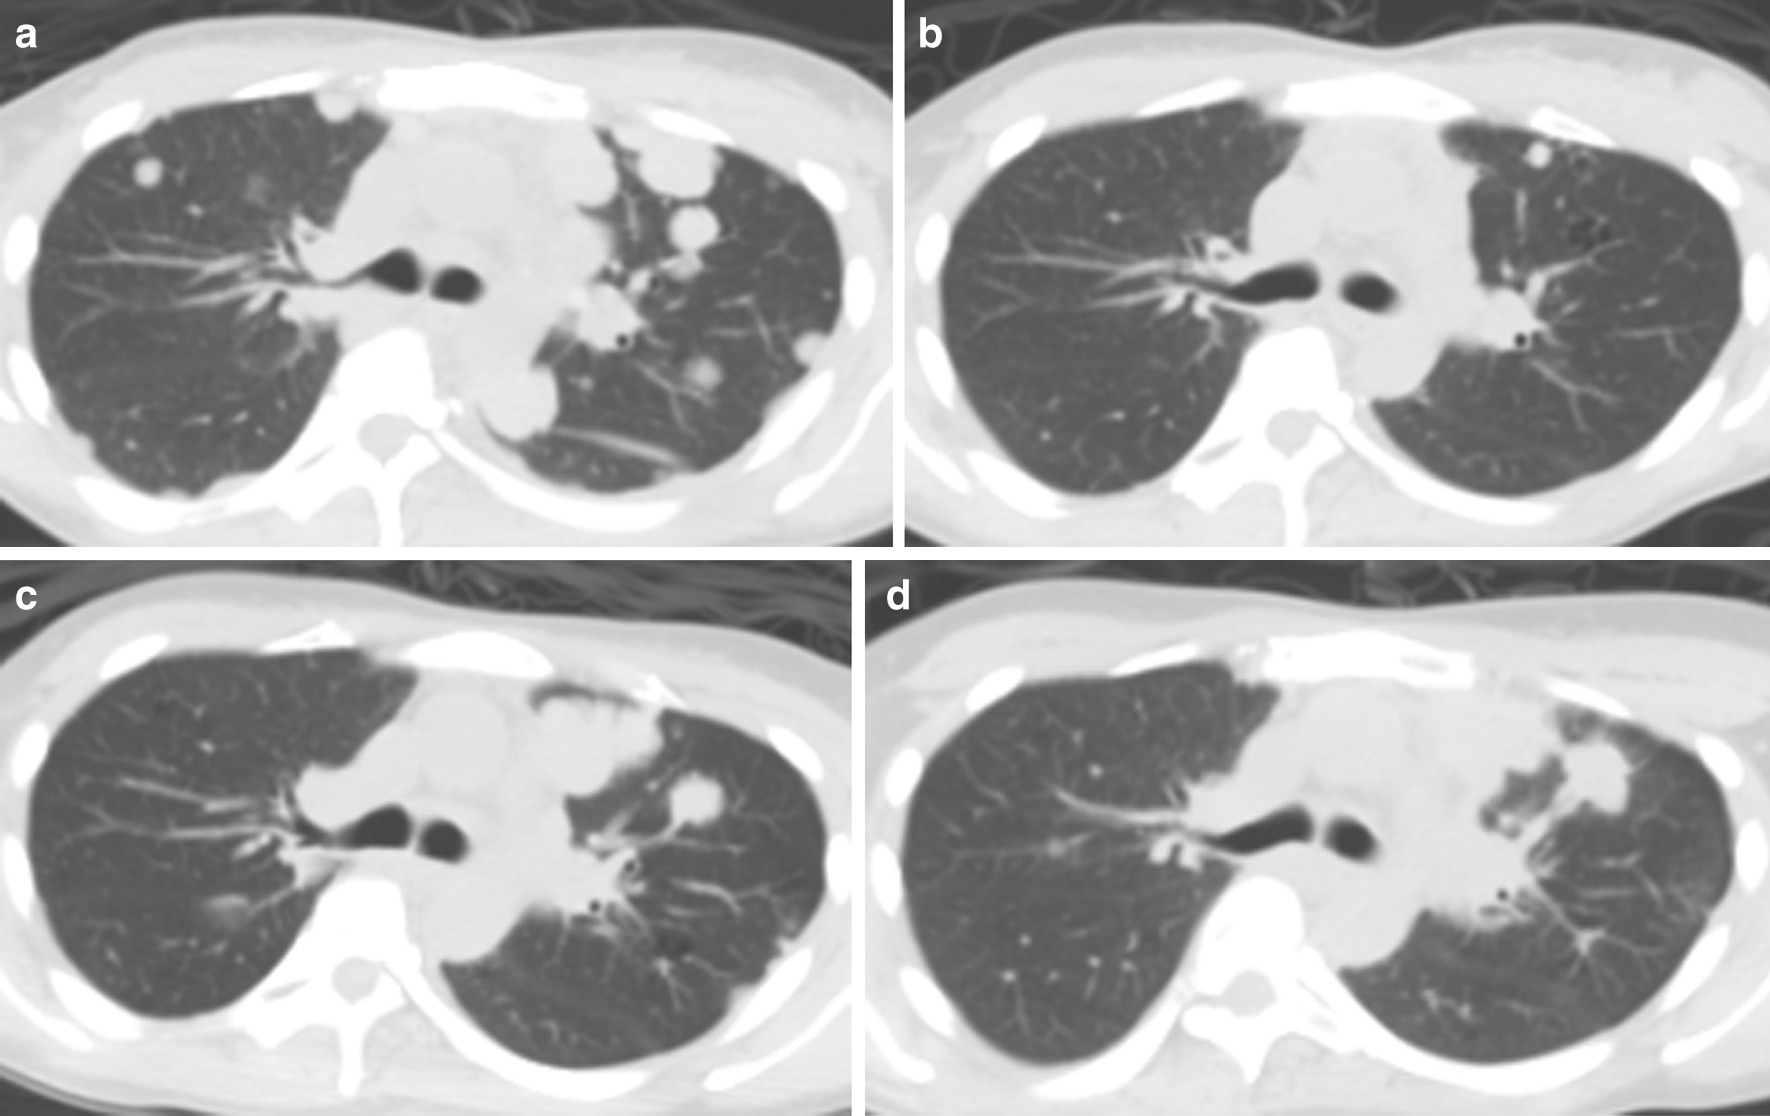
Serial positron emission tomography with integrated computed tomography images showing response to lung metastases before and after pembrolizumzb treatment, interruption and rechallenge during and after anti-tuberculosis (TB) therapy. a Multiple bilateral lung metastases before pembrolizumab treatment. b Marked reduction in size and number of lung metastases after pembrolizumab treatment. c Enlarging lung metastases after pembrolizumab interruption due to TB reactivation. d Tumour shrinkage after re-challenge with pembrolizumab
